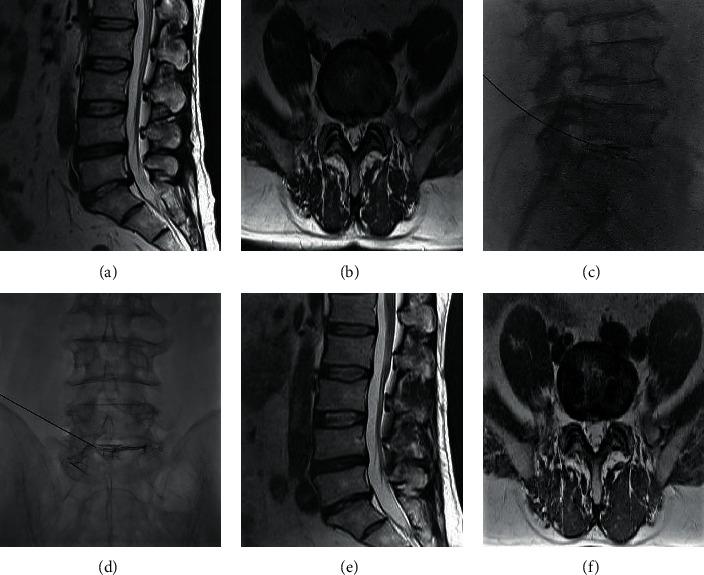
Low-dose CCNL combined with RF for LDH. (a, b) Patients lumbar MRI at preoperatively: L5S1 disc herniated. (c, d) Intraoperative X-ray radiography: contrast agent diffuses during operation. (e, f) Patients lumbar MRI at 10 years postoperatively: the herniation of L5S1 disappeared.
